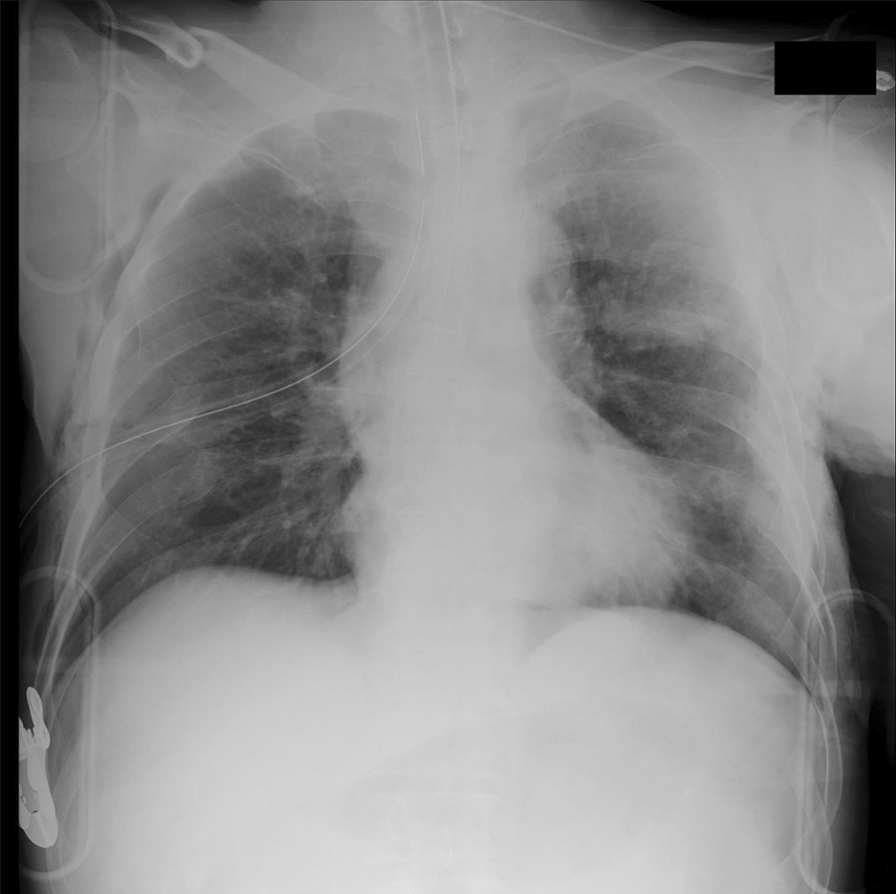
Chest X-ray at primary survey
